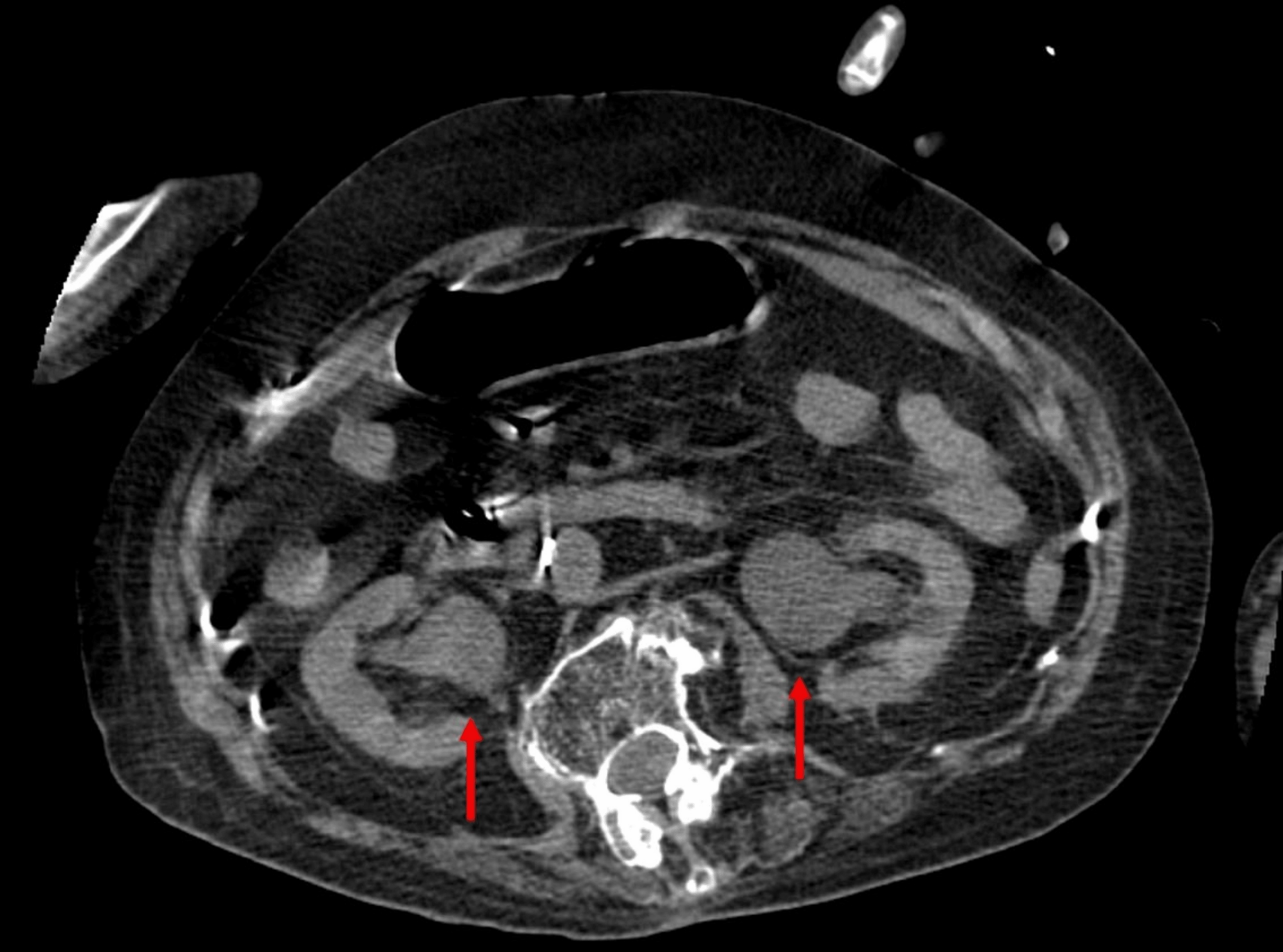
Abdominal CT Hydronephrosis was present in both kidneys. The arrows indicate an enlarged renal pelvis in both kidneys.
